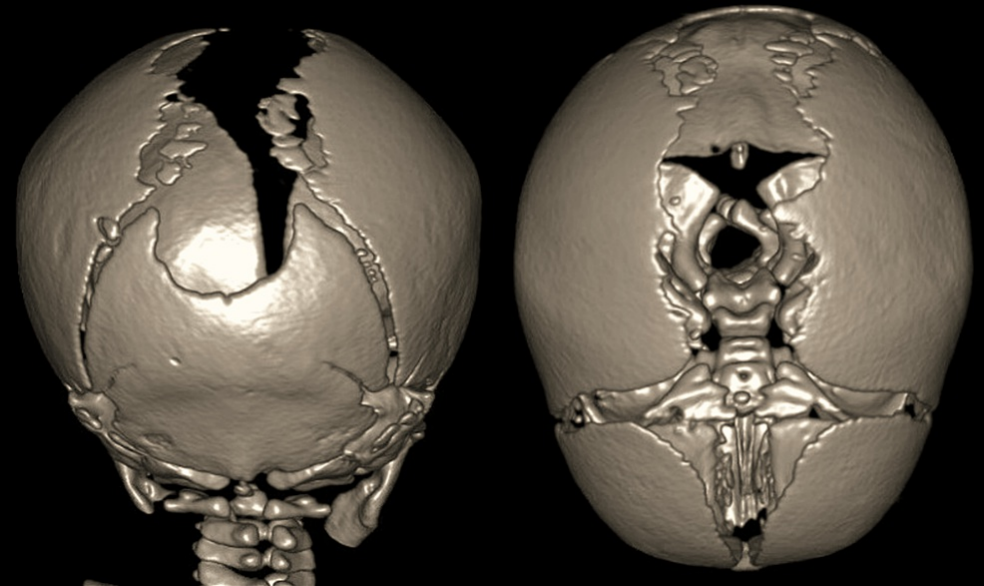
CT scan of the head The 3D reconstruction from a CT scan reveals extensive defects extending posteriorly from the anterior fontanelle.
